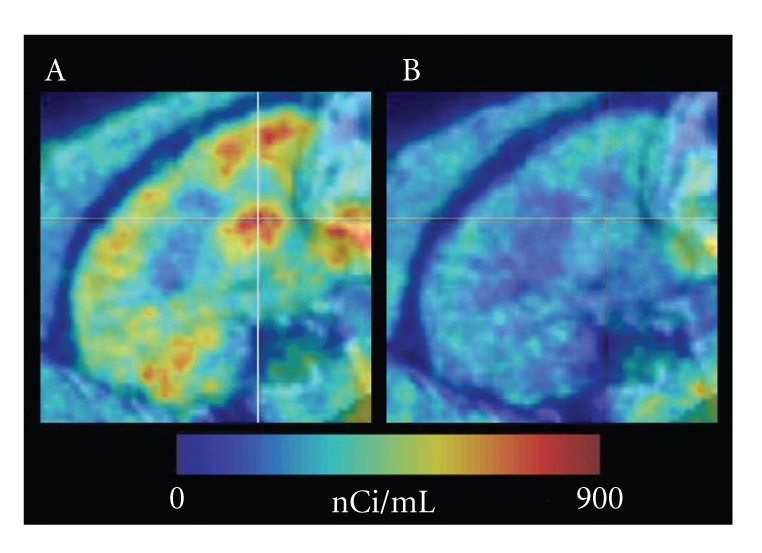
Averaged saggital baboon brain PET images of [11C]JHU75528 scans, displayed on aligned MRI images. Lines
